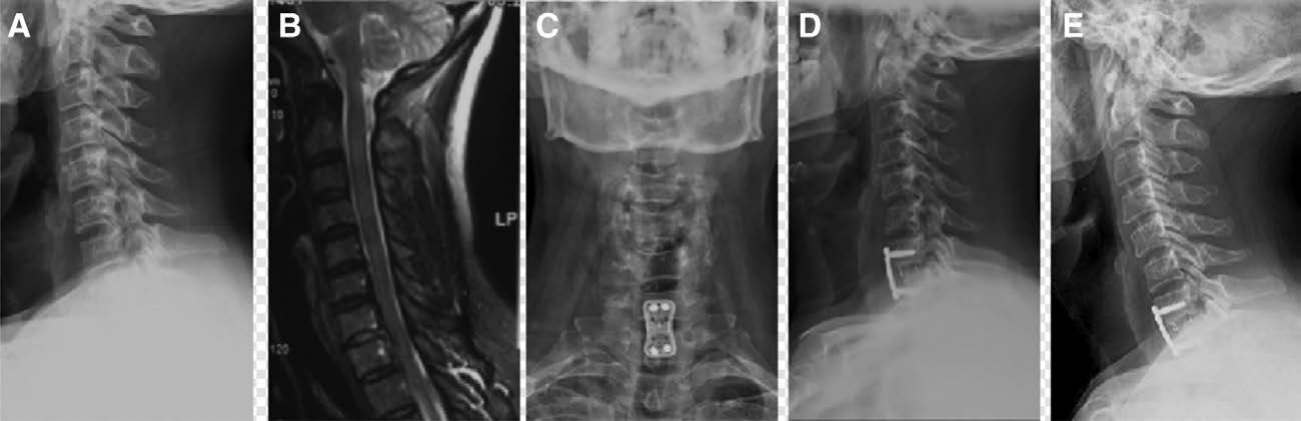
Case 1: (A) Lateral radiograph of cervical vertebra indicates that the physiological curvature of cervical vertebra becomes straight; (B) MRI sagittal plane of cervical vertebra shows I° spondylolisthesis of C7 vertebral body and protrusion of C7/T1 intervertebral disc; (C and D) the radiography showed that the internal fixation position was good after anterior decompression and fusion; (E) postoperative lateral radiography showed bone fused well at 12 months after operation.
